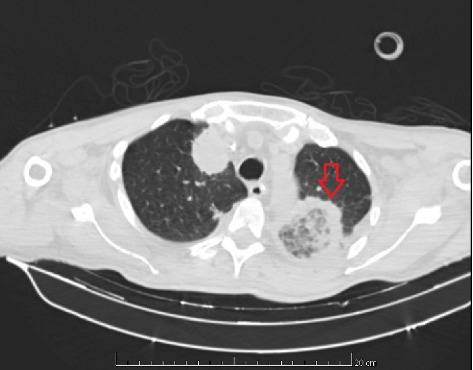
Chest CT scan showing an inverted halo sign on the left upper lobe (red arrow) characterized by a central ground-glass opacity surrounded by a peripheral rim of consolidation. This radiologic finding is often associated with a range of pulmonary conditions, including angioinvasive fungal infections (e.g., invasive aspergillosis and mucormycosis), organizing pneumonia, and pulmonary infarction.
